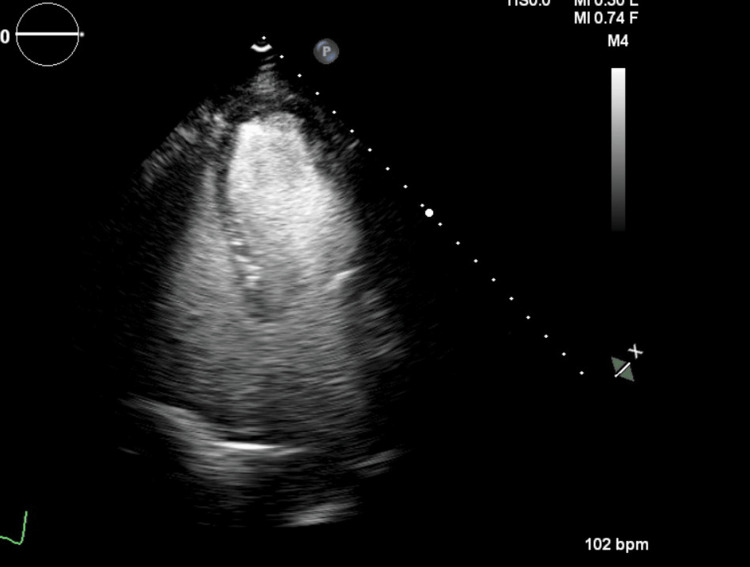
Transthoracic echocardiogram during diastole
